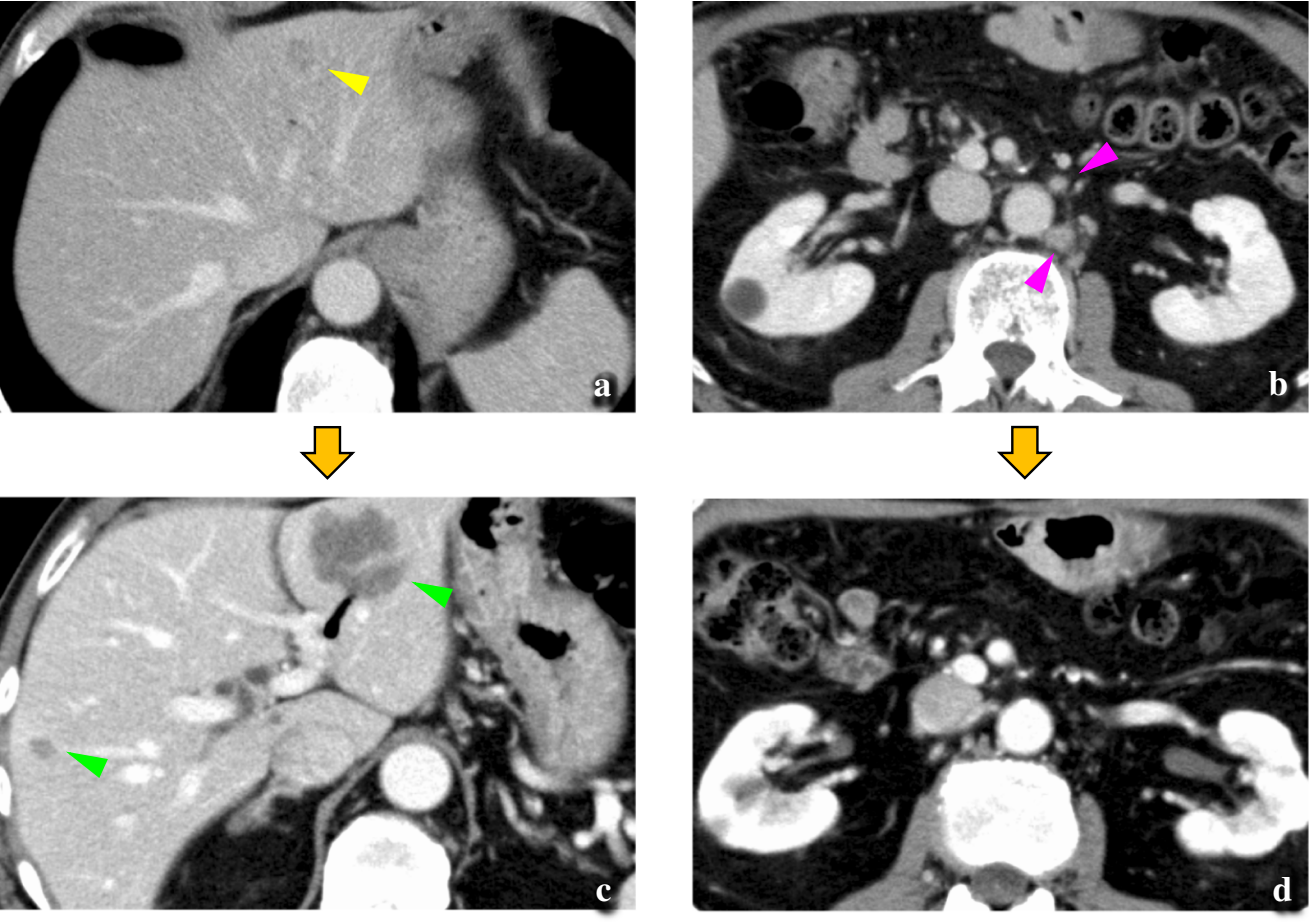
Computed tomography. Liver metastasis in segment 3 (a yellow arrowhead) and swollen para-aortic lymph nodes were identified (b pink arrowheads) 12 months after laparoscopic pancreatoduodenectomy. After six courses of chemotherapy, the liver deposits progressed in segments 3 and 5 (c green arrowheads), while the lymph nodes regressed (d)
